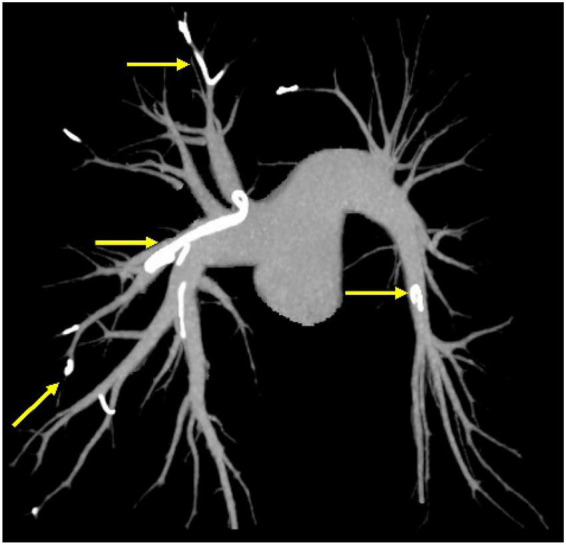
Computed tomography pulmonary angiography (CTPA) of the pulmonary arteries showed that striated dense shadows in the lungs persisted after the patient’s treatment.
